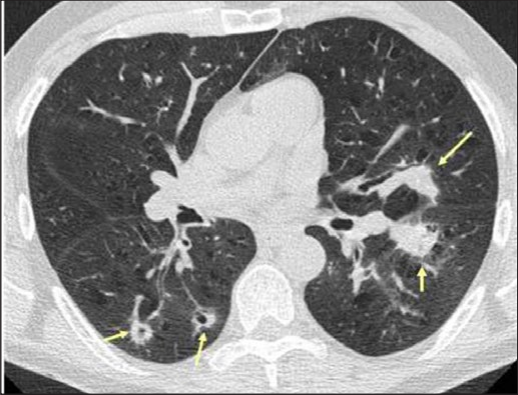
The patient returned with cough and malaise a year later. CT showed an increase in the size of the nodules as well as cavitation. Bronchial lavage produced acid-fast bacilli, later proved to be mycobacterium avium intracellulare.
